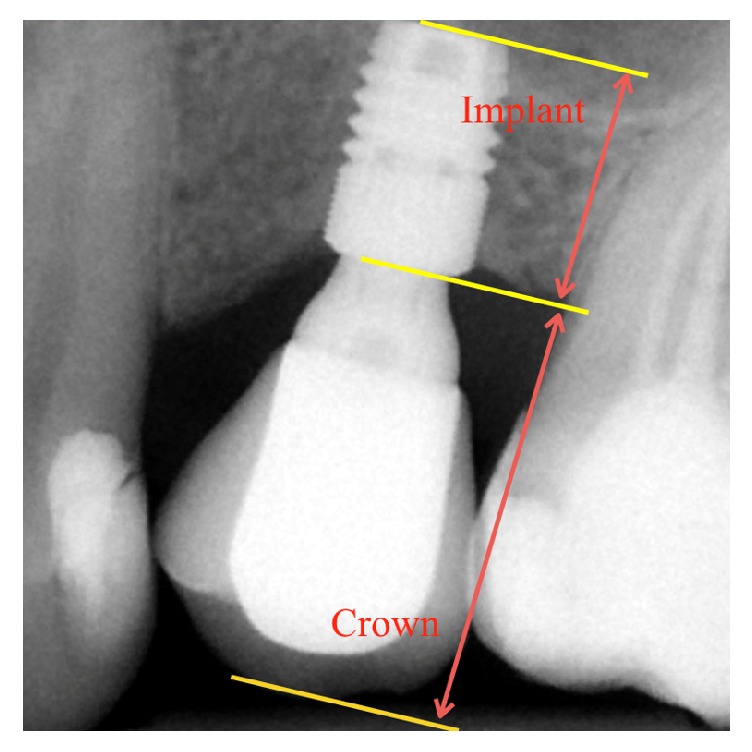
Periapical digital radiograph of a short implant (Astra Tech implant system™ OsseoSpeed TX 4.0 S; Ø4 mm, 6 mm long). The *C*/*I* ratio measurement method is presented. Radiological status 36 months after implant placement.
